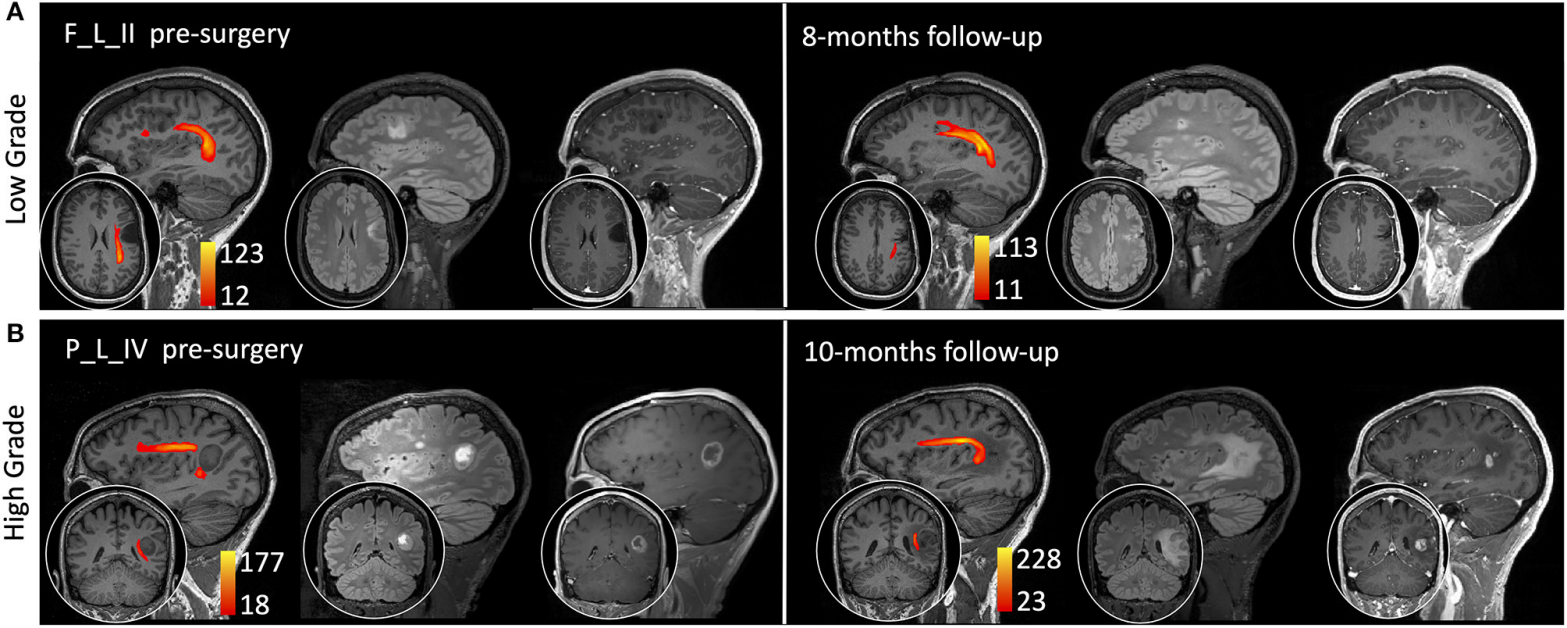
Sagittal view on the tumor side and coronal or axial focus of T1-weighted, fluid-attenuated inversion recovery (FLAIR) T2-weighted, and T1-weighted images after gadolinium administration (0.1 mm/kg). The arcuate fasciculus tractography is superimposed on T1-weighted images (colormap red to yellow indicating increasing fiber reconstruction probability). (A) A low-grade glioma patient pre-surgery (left side) and 8 months post-surgery (right side). (B) A high-grade glioma patient pre-surgery (left side) and 10 months post-surgery (right side). Patients are labeled according to the tumor's (lobe)_(hemisphere)_(grade) classification: parietal (P) and frontal (F) lobe; and left (L) hemisphere.
